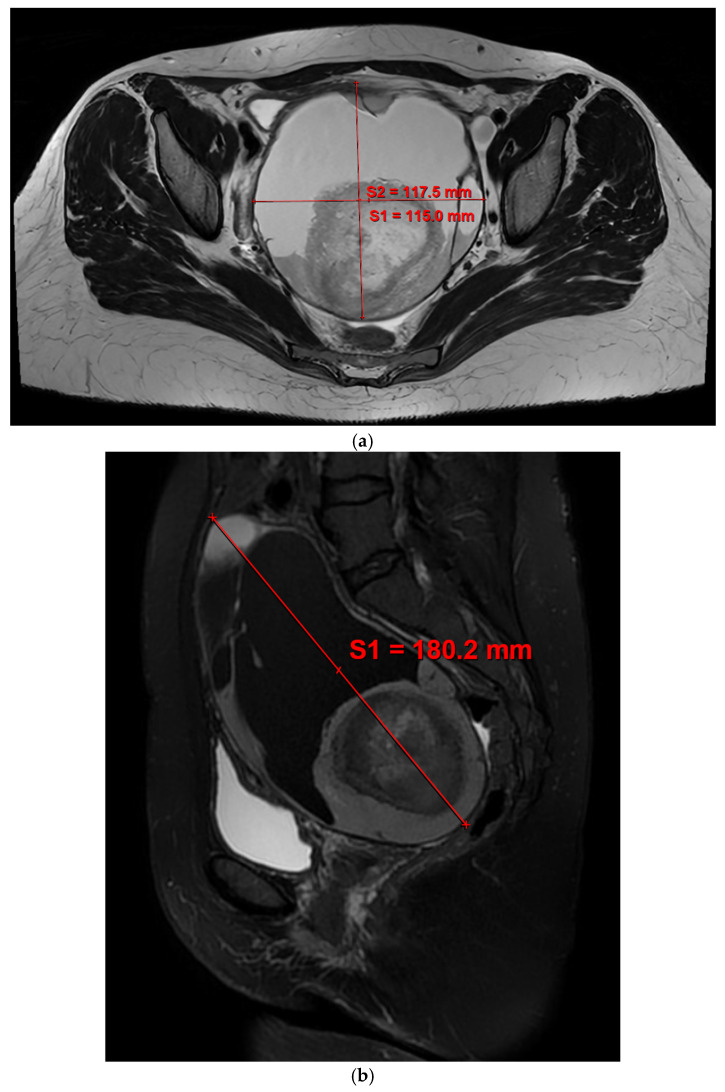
MRI of the ovarian mass: (a) transverse view, (b) sagittal view.
